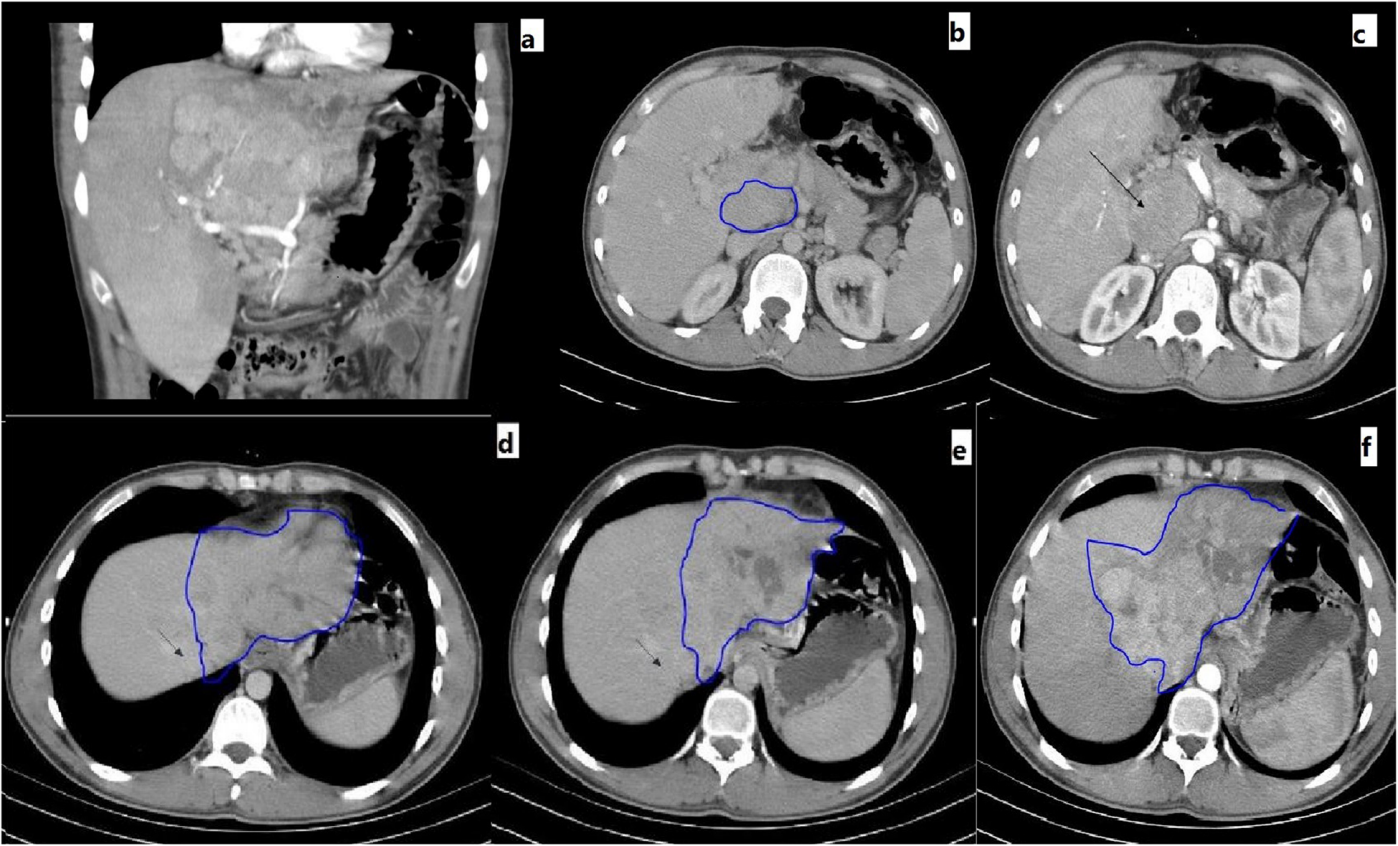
CT scan images of the involved liver. a HCC involving the liver from a coronal view. b, c Multiple lymph nodes in the hilum of the liver (marker shows the largest lymph node). d, e Tumor involvement of the left and middle hepatic veins and neighboring of the right hepatic vein (arrow). f HCC of the left lobe of the liver with involvement of retrohepatic IVC and caudate lobe
